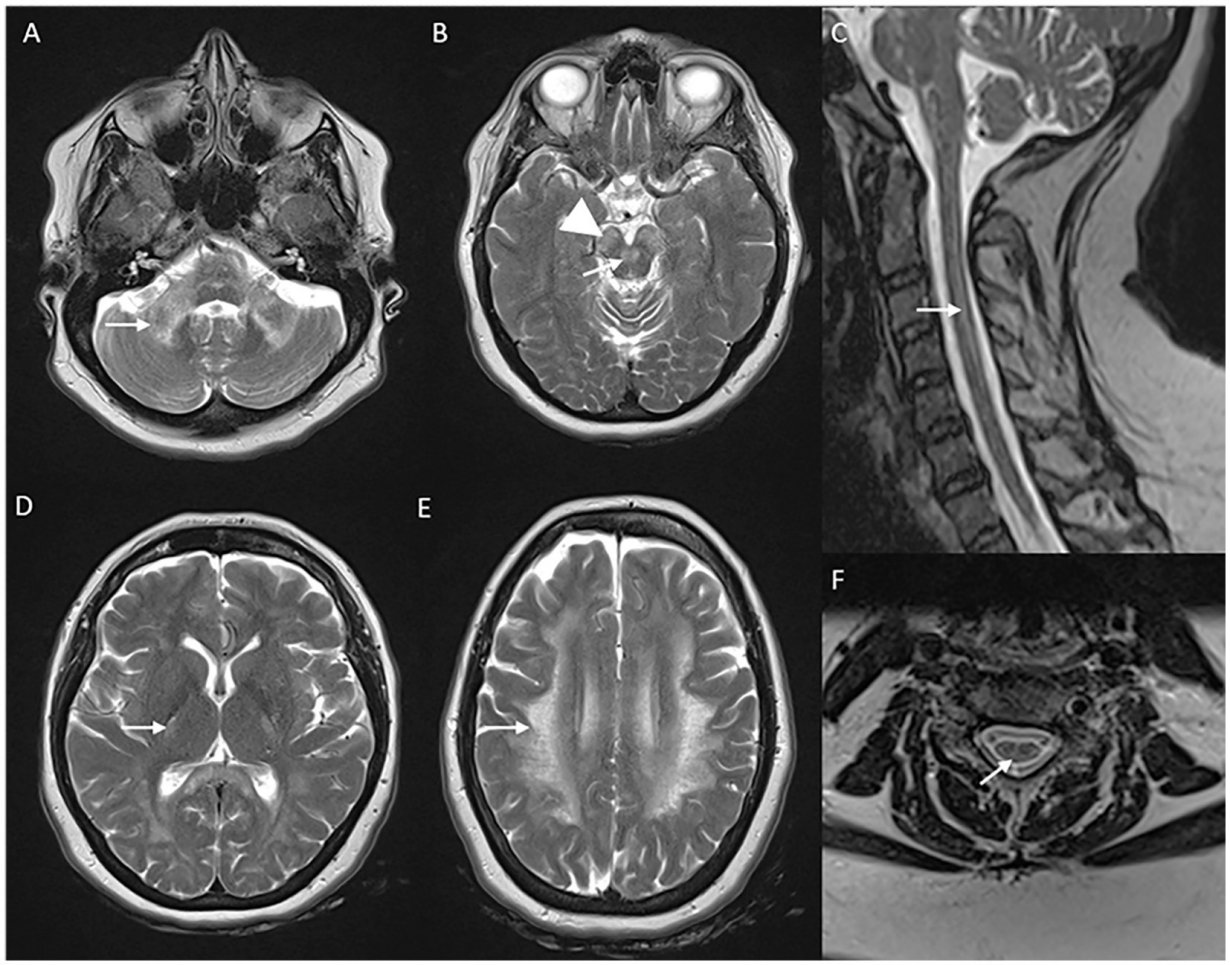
Neuroimaging in *LMNB1*-related autosomal-dominant leukodystrophy (autosomal-dominant adult-onset demyelinating leukodystrophy). MRI brain T2W axial section revealing symmetric signal changes in middle cerebellar peduncles (A), medial longitudinal fasciculus (arrow in B), crus cerebri [arrowhead in (B)], posterior limb of the internal capsule (D), and confluent, extensive subcortical and deep white matter changes in high frontoparietal regions. T2W sagittal (C) and axial (F) spine images show long segment signal changes along the cervical cord with the involvement of the lateral and dorsal columns.
